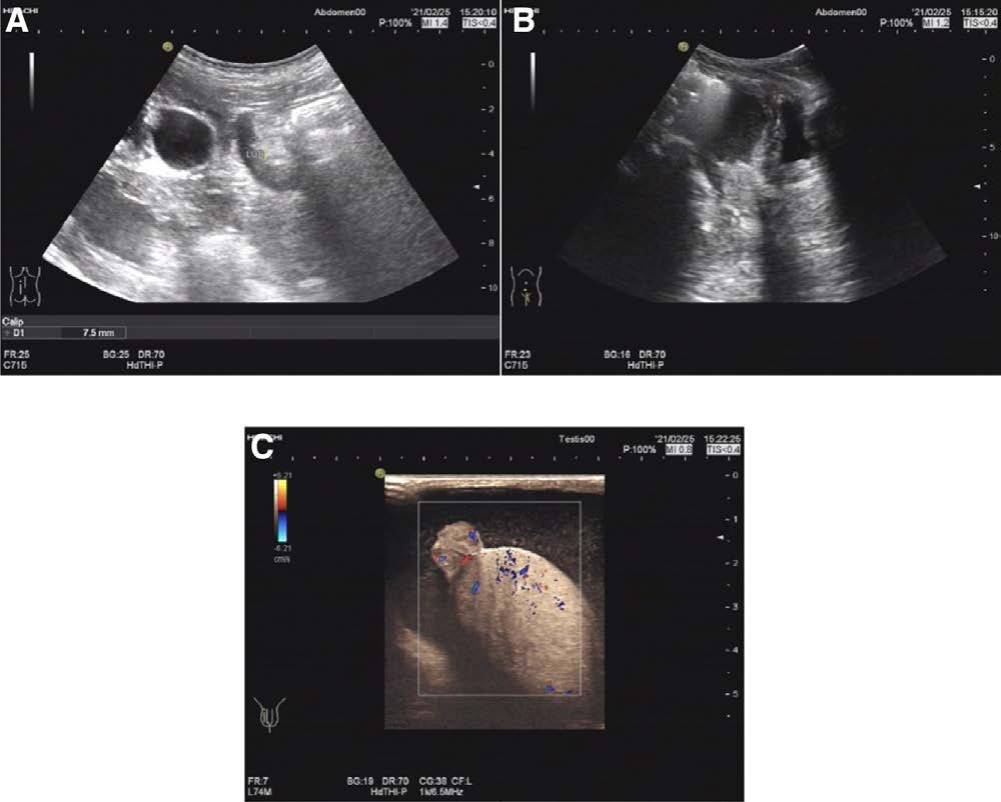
Color ultrasonography of scrotum.
